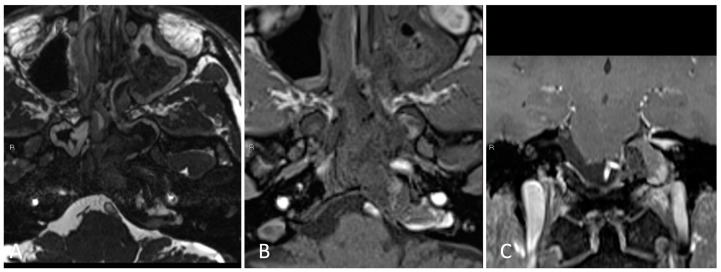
Postoperative imaging demonstrating decompression of a petrous apex cholesterol granuloma. (A) Axial T2 high-resolution constructive interference steady state (CISS) T2-weighted MRI. (B) Axial T1 post-contrast MRI. (C) Coronal T1 post-contrast MRI.
